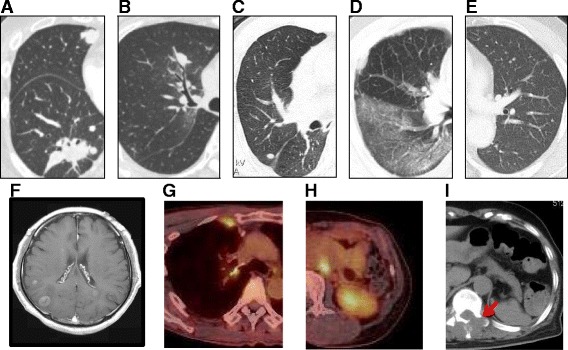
Representative cases for the assessment of re-biopsy feasibility Progressive or relapsing sites were evaluated for the feasibility of re-biopsy according to the criteria shown in Table 1. a Lung nodule (long diameter ≥ 2 cm) with a responsive bronchus leading to the center of the lesion (category A for trans-bronchial biopsy [TBB]). b Lung nodule adjacent to the nearest bronchus (category A for TBB). c Lung nodule not reaching a responsive bronchus but accessible by CT-guided needle biopsy (CTNB) (long diameter < 1 cm and ≤5 cm distant from the thoracic wall category B for CTNB). d Diffuse pulmonary infiltration presenting a lymphangitic distribution (category A for trans-bronchial lung biopsy [TBLB]). e Small pulmonary metastases (not feasible or extremely difficult for TBB or CTNB; category C). f Brain metastases (surgical resection required; category C). g Solitary pleural metastasis (long diameter ≤ 1 cm; category B for CTNB). h Para-aortic lymph node metastasis, front and adjacent to the abdominal aorta (CTNB is highly unsafe; category C). i Bone metastasis with extra-osseous invasion (category A for CTNB)
